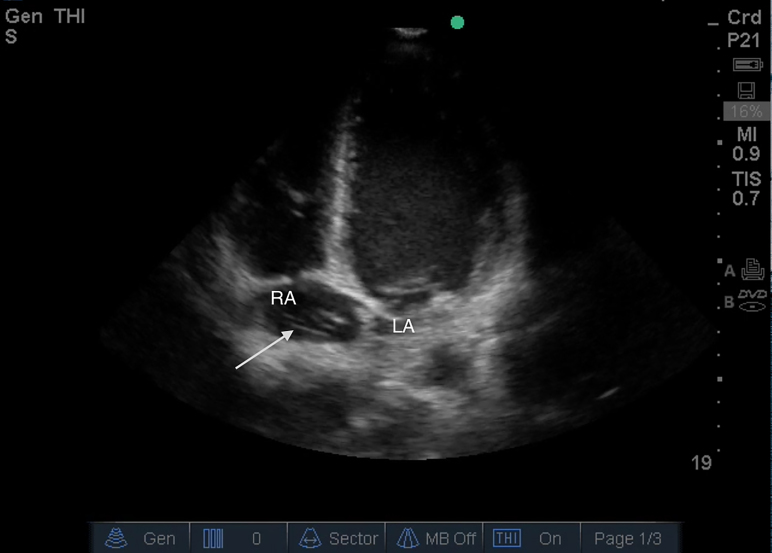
Apical four chamber view demonstrating a right atrial Chiari Network (arrow). Right Atrial Thrombus or Chiari Network? *Fredericks et al.*
